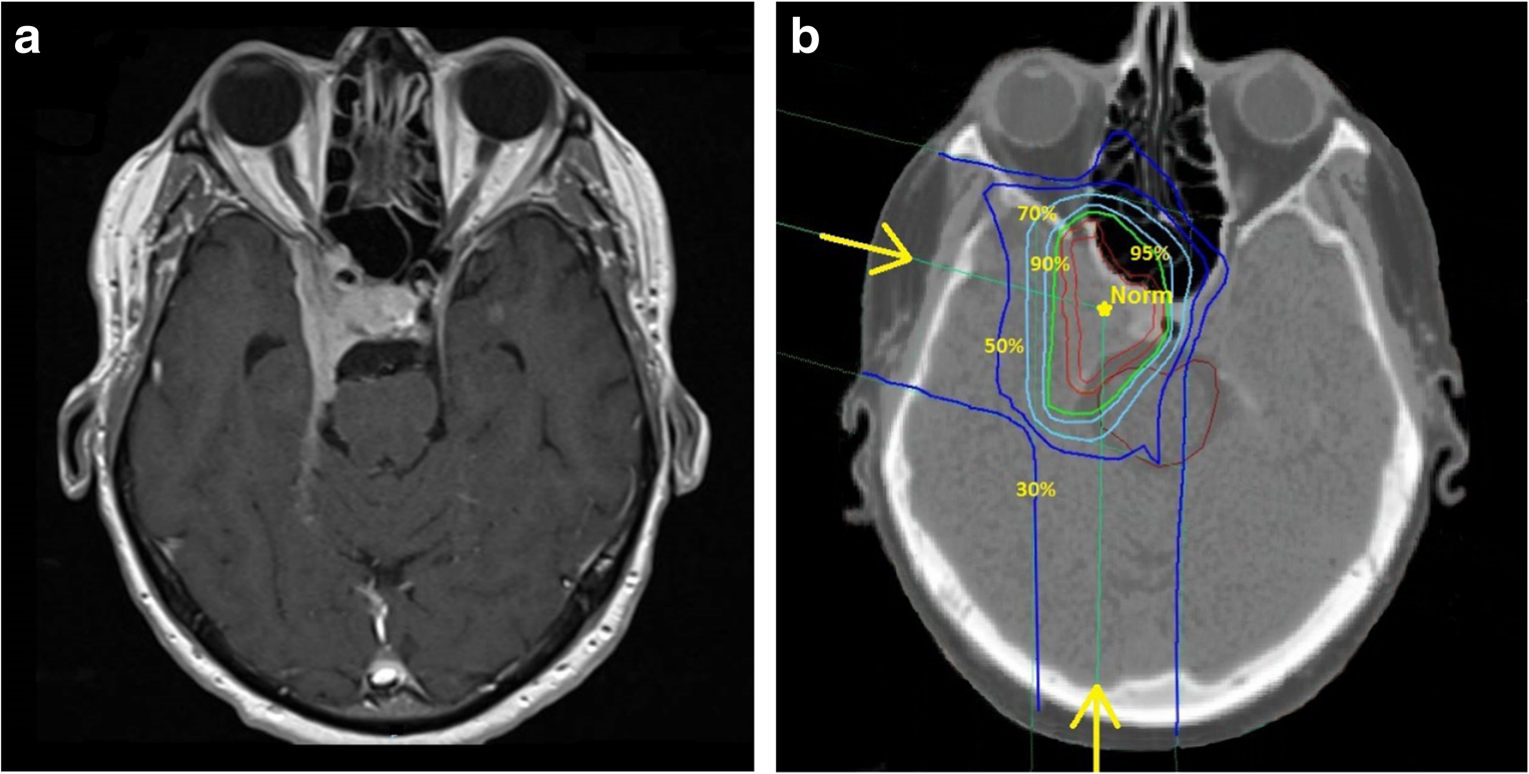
a MRI scan of right-sided cavernous sinus meningioma. b CT scan and treatment plan in section corresponding closely to the MRI scan in a. The dose prescribed to the target in the ICRU reference point is indicated as *Norm*. The *isodose lines* shown are 30, 50, 70, 90 and 95% of the prescribed dose
